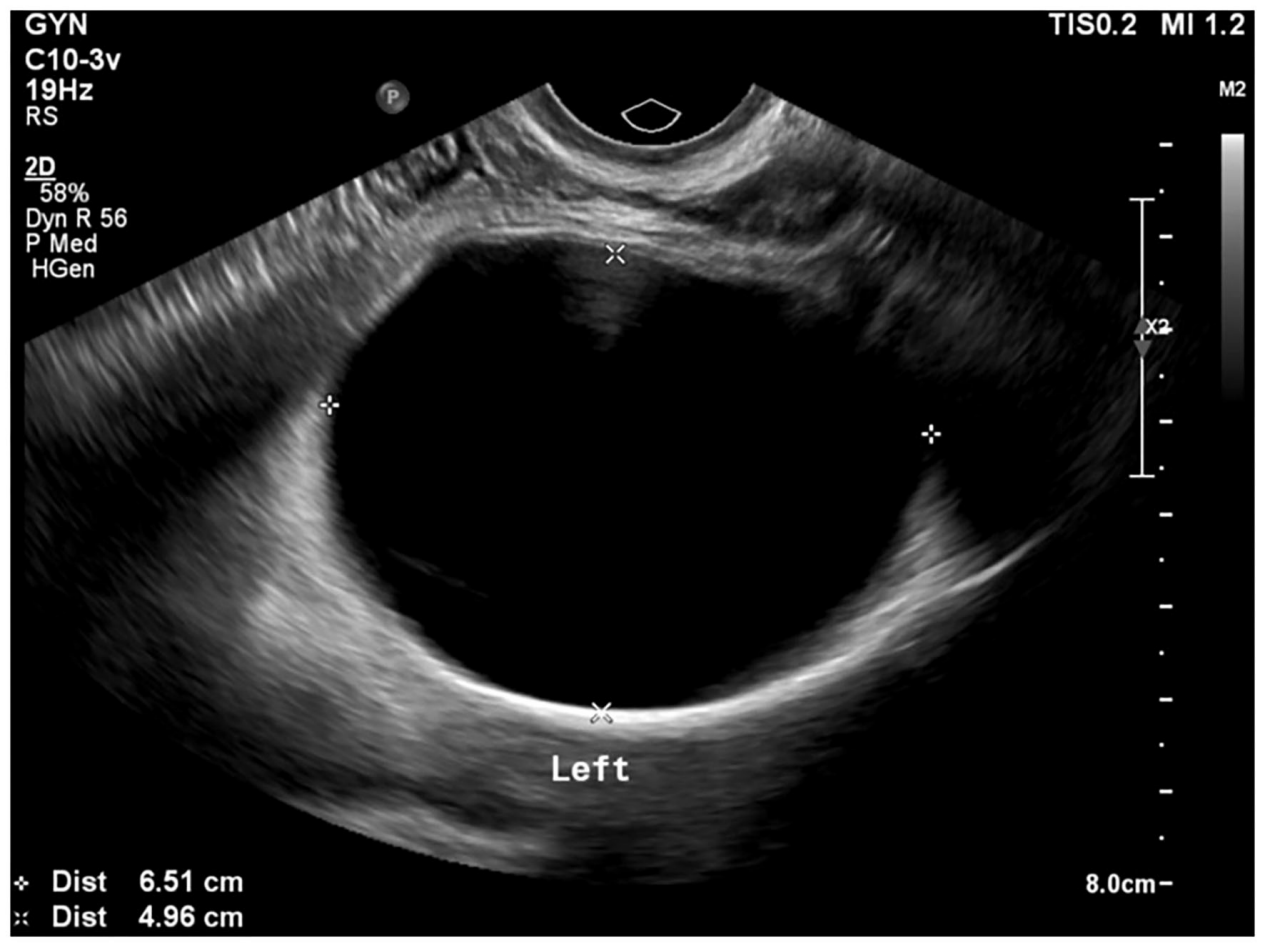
Pelvic ultrasonography shows a left 6.51 × 4.96 cm ovarian cyst.
